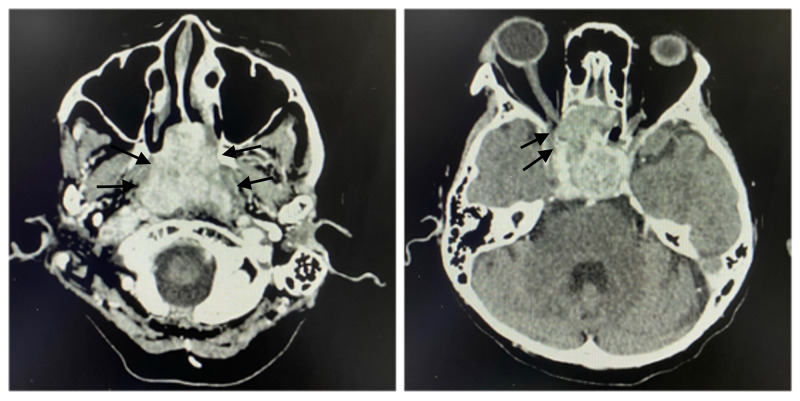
CT-scan shows a heterogenous enhancing soft tissue mass within nasopharynx, obliterating the fossa of Rosenmuller and torus tobarius bilaterally, with infiltration of skull base and right cavernous sinus.
